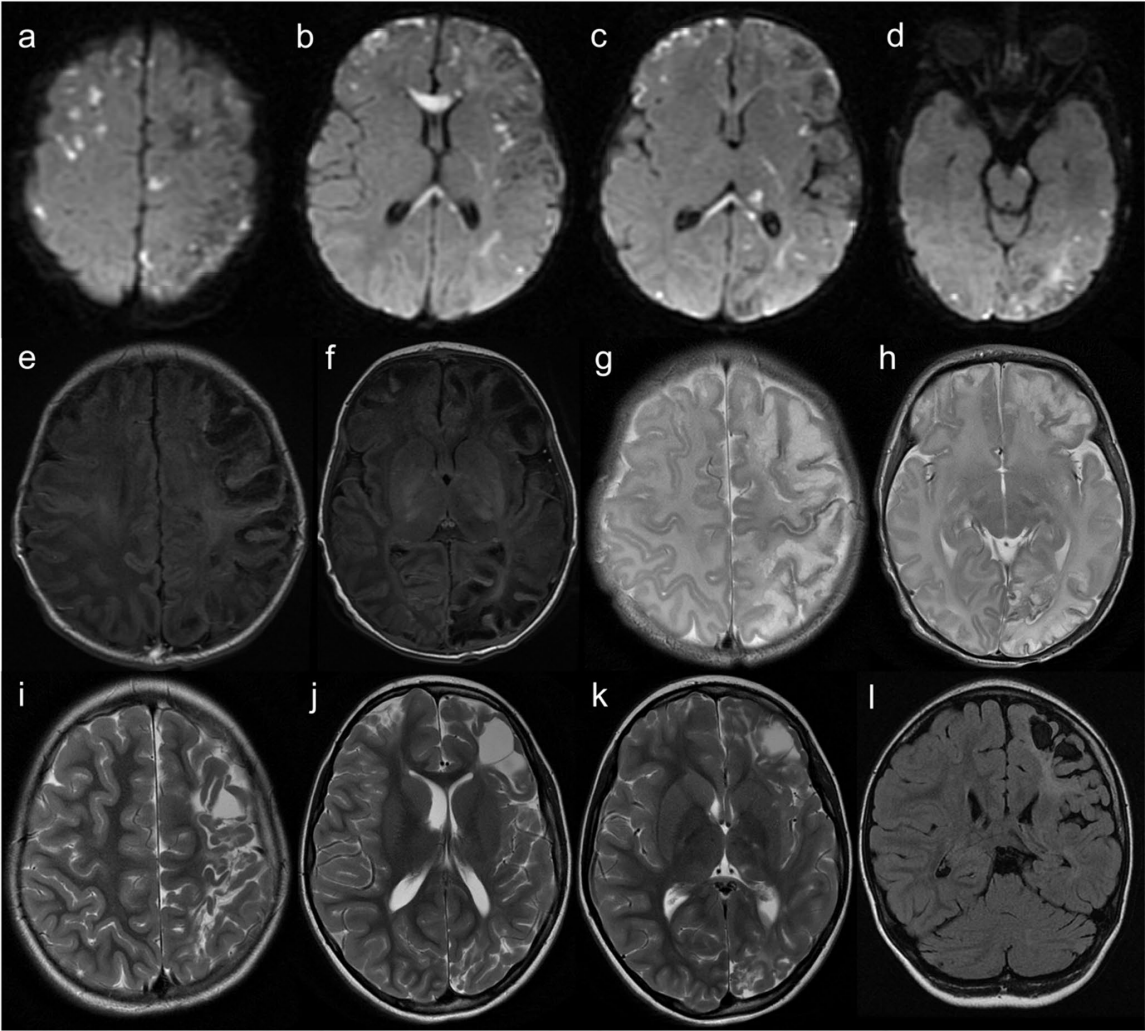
Incontinentia pigmenti. A 12-day-old female patient with seizures and a vesiculobullous skin rash. (a to d) Axial b1000 DWI demonstrates multiple hyperintense cortical and subcortical lesions (corresponding low signal on ADC maps is not shown) in the cerebral hemispheres, corpus callosum, left thalamus, and ipsilateral posterior limb of the internal capsule and cerebral peduncle. (e and f) Axial T2 and (g and h) FLAIR images reveal extensive subcortical white matter hyperintensities, predominantly in the left cerebral hemisphere. (i, j, and k – axial T2; l – coronal FLAIR) Follow-up brain MRI after 7 years shows progression to left cerebral hemiatrophy and white matter cavitation
